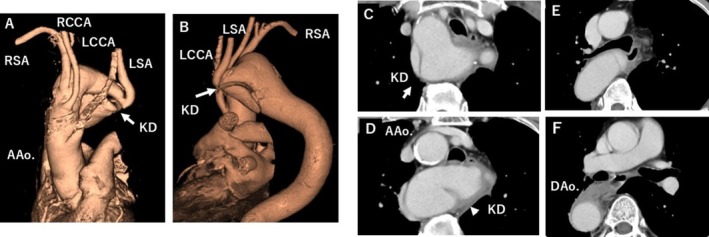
Computed tomography (CT) in Case 1. Demonstrating aortic dissection. (A, B) Three‐dimensional volume‐rendered CT images from anterior and posterior views, respectively, showing a right aortic arch with a Kommerell's diverticulum (KD, arrows). (C–F) Axial CT images showing the KD and the intimal flap of the aortic dissection (arrowhead in D and E). Abbreviations: AAo., ascending aorta; DAo., descending aorta; RCCA, right common carotid artery; LCCA, left common carotid artery; RSA, right subclavian artery; LSA, left subclavian artery.
